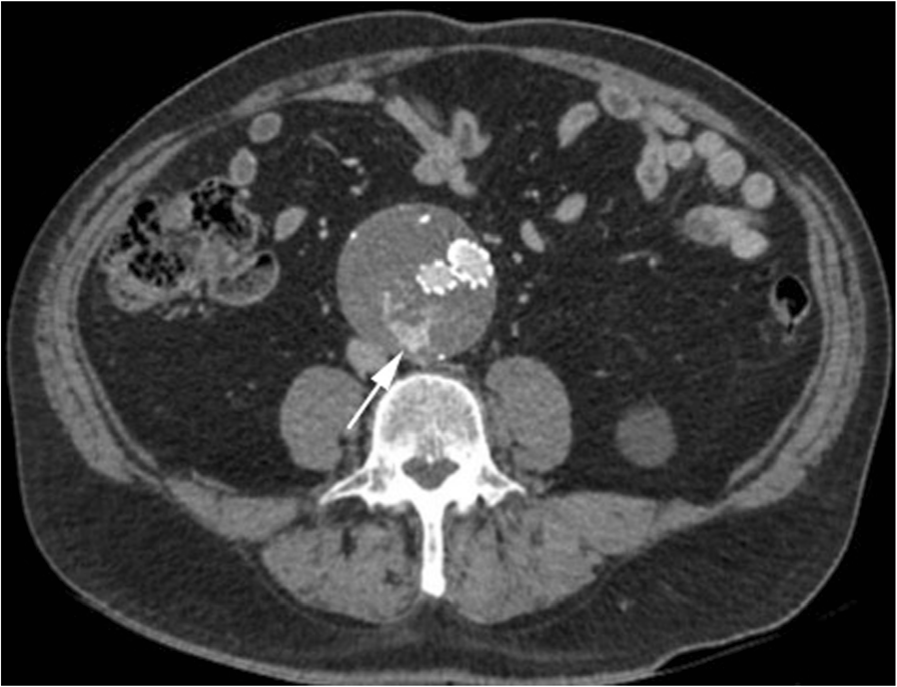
Axial computed tomography angiography (CTA) through the lower abdomen shows an abdominal aortic aneurysm with a T2E (white arrow) arising from an adjacent lumbar artery
